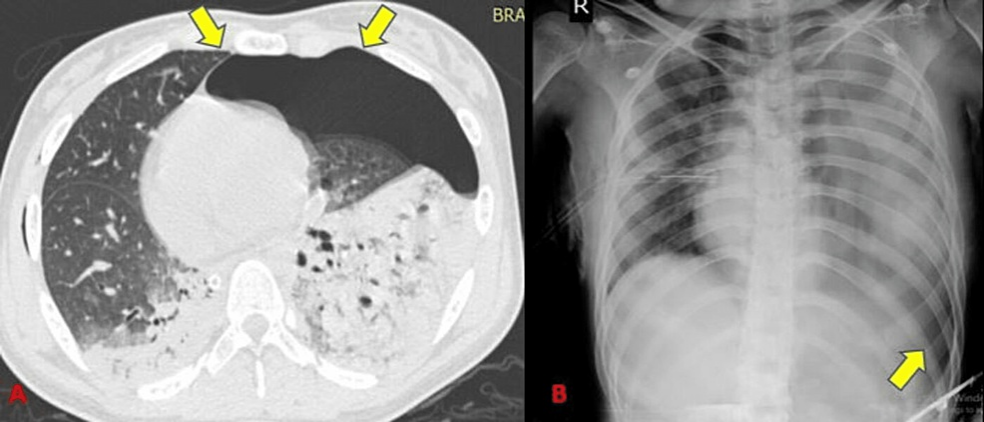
Tension pneumothorax. There is contralateral shift of mediastinum on CT and deep sulcus sign on radiograph.
